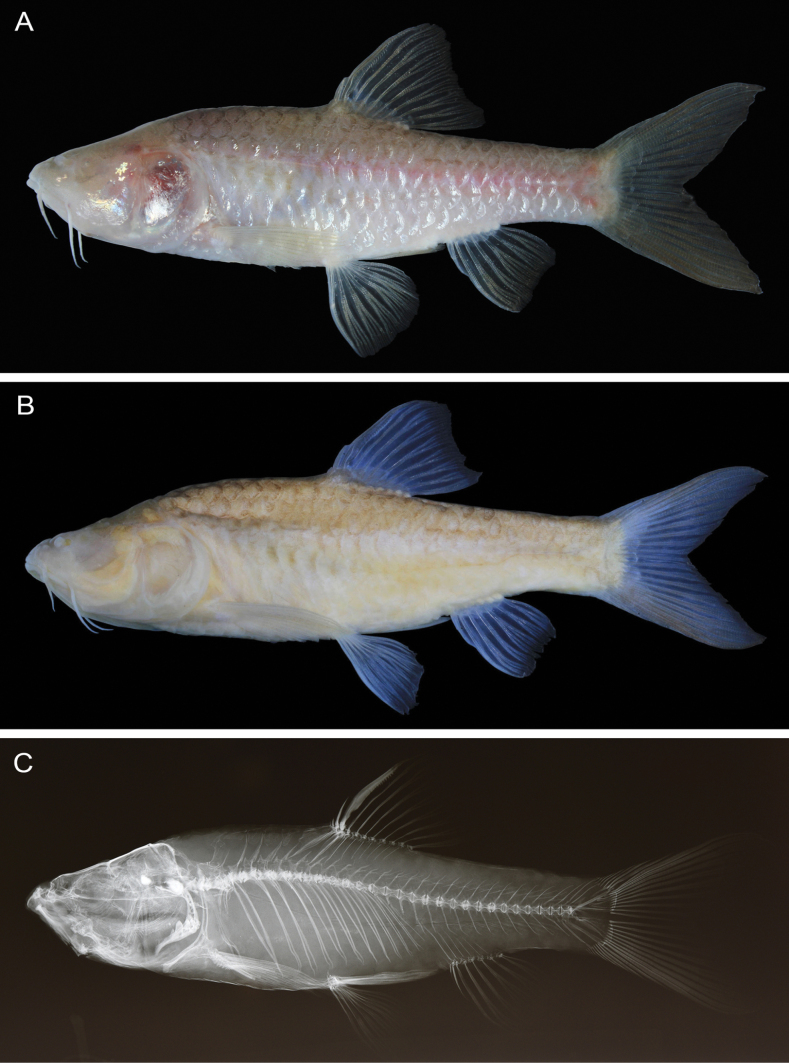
Barbodesklapanunggalensis* sp. nov., MZB.26657, holotype, 63.8 mm SL A fresh B preserved C radiograph.
